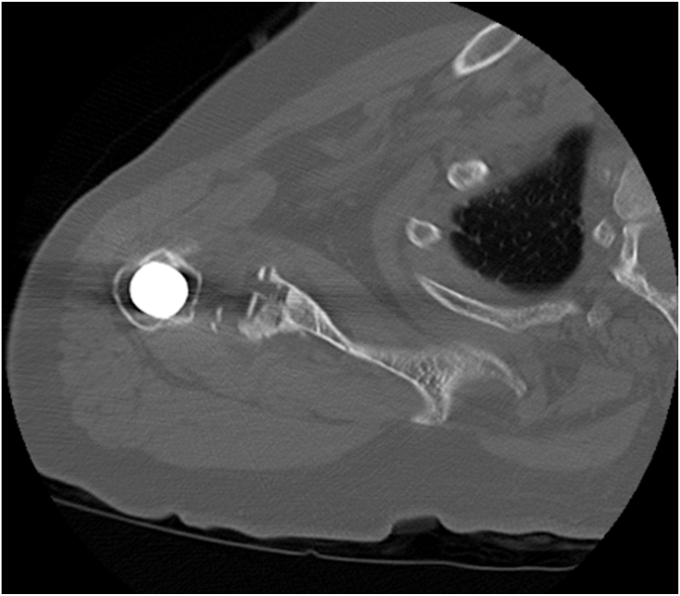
Preoperative axial *CT* demonstrating a glenoid defect suggesting aseptic loosening of prior total shoulder arthroplasty. *CT*, computed tomography.
